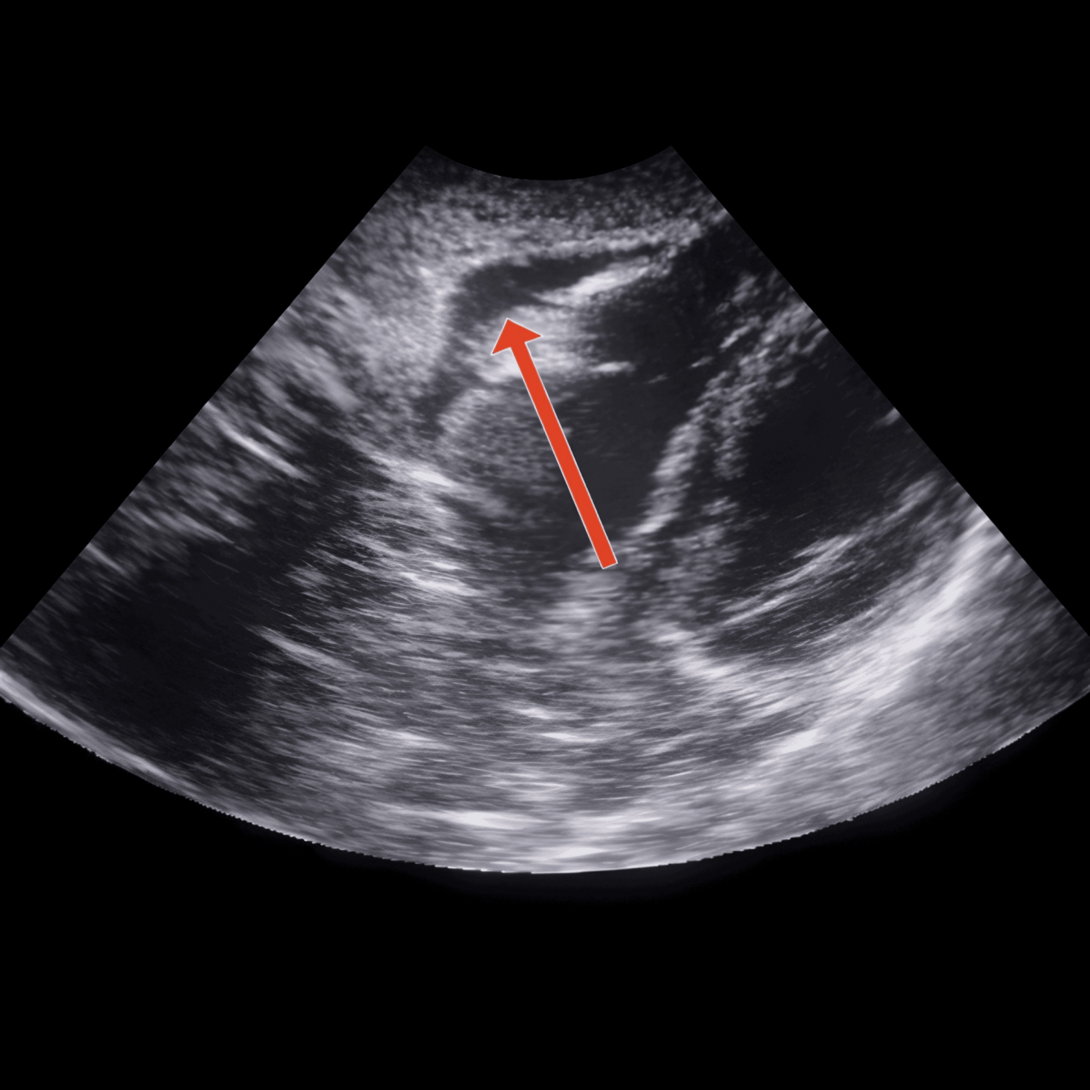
Pericardial fluid seen on EFAST scan EFAST, extended focused assessment with sonography in trauma
